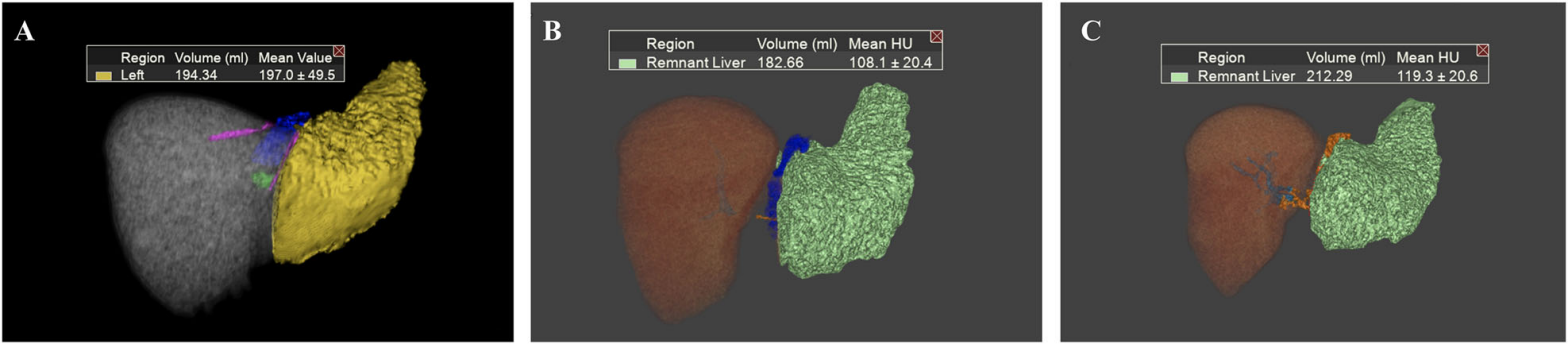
Unsatisfied liver regeneration in the interval stage. A 36-year-old male patient, who did not undergo ALPPS stage 2 surgery due to insufficient liver volume. A three-dimensional image of the preoperative MRI shows the liver volume of the left lobe is 194.34 mL. B Three-dimensional image of the CT in 7 days after the ALPPS stage 1 shows no significant liver regeneration of the left lobe; the volume is 182.66 mL. C Three-dimensional image of the CT in 21 days after the ALPPS stage 1 shows still slow liver regeneration of the left lobe, the volume is 212.29 mL (16.19% of the standard liver volume)
